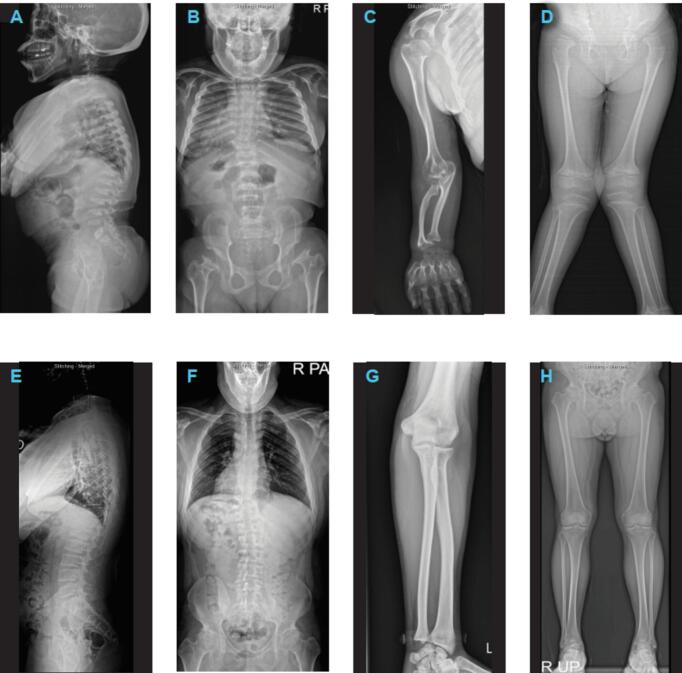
Radiographs of patient 12 (severe type) and patient 1 (attenuated). Patients 12: A, lateral (17.3 years); B, anterior (17.3 years); C, forearm (17.3 years), D, whole leg (6 years old prior to hemiephiphysiodesis). Patient 1: E, lateral (28.1 years), F, anterior (28.1 years); G, forearm (28.1 years); H, whole leg (28.1 years).
